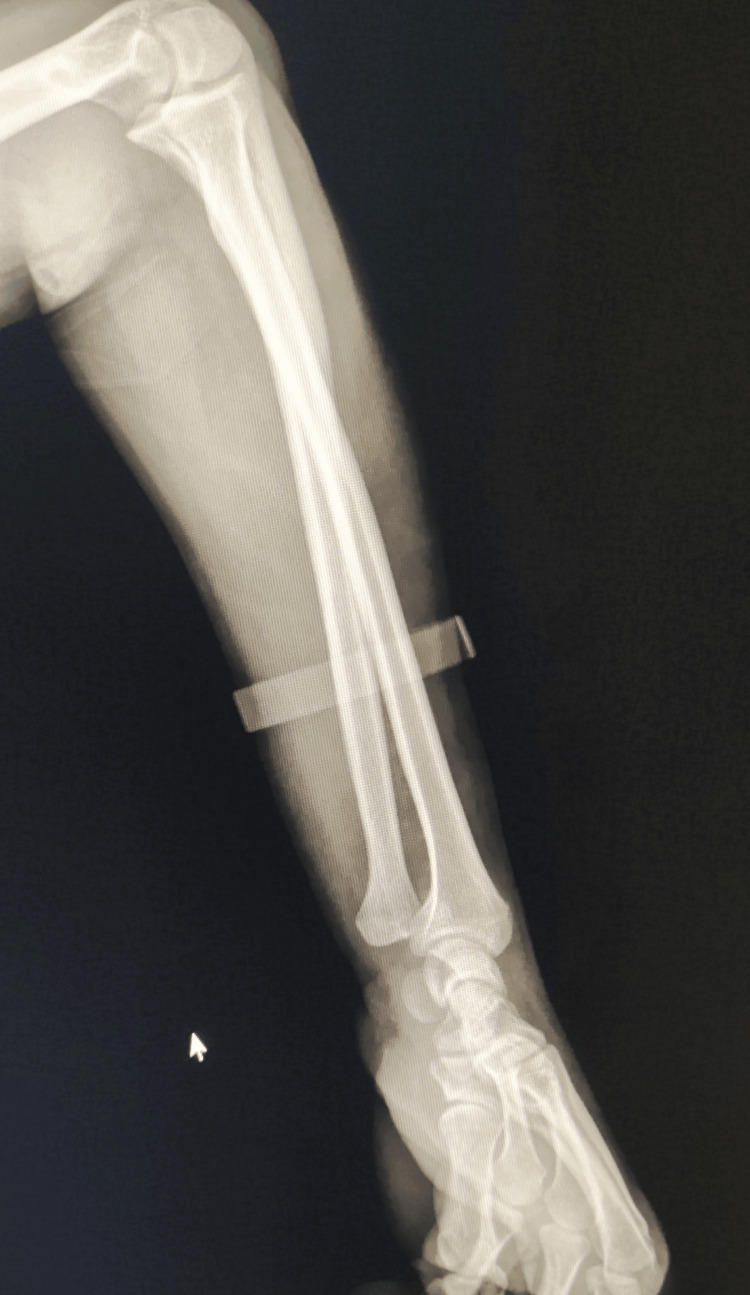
Lateral wrist X-ray Oblique lateral wrist radiograph with inadequate visualization of the distal radioulnar joint due to patient discomfort.
